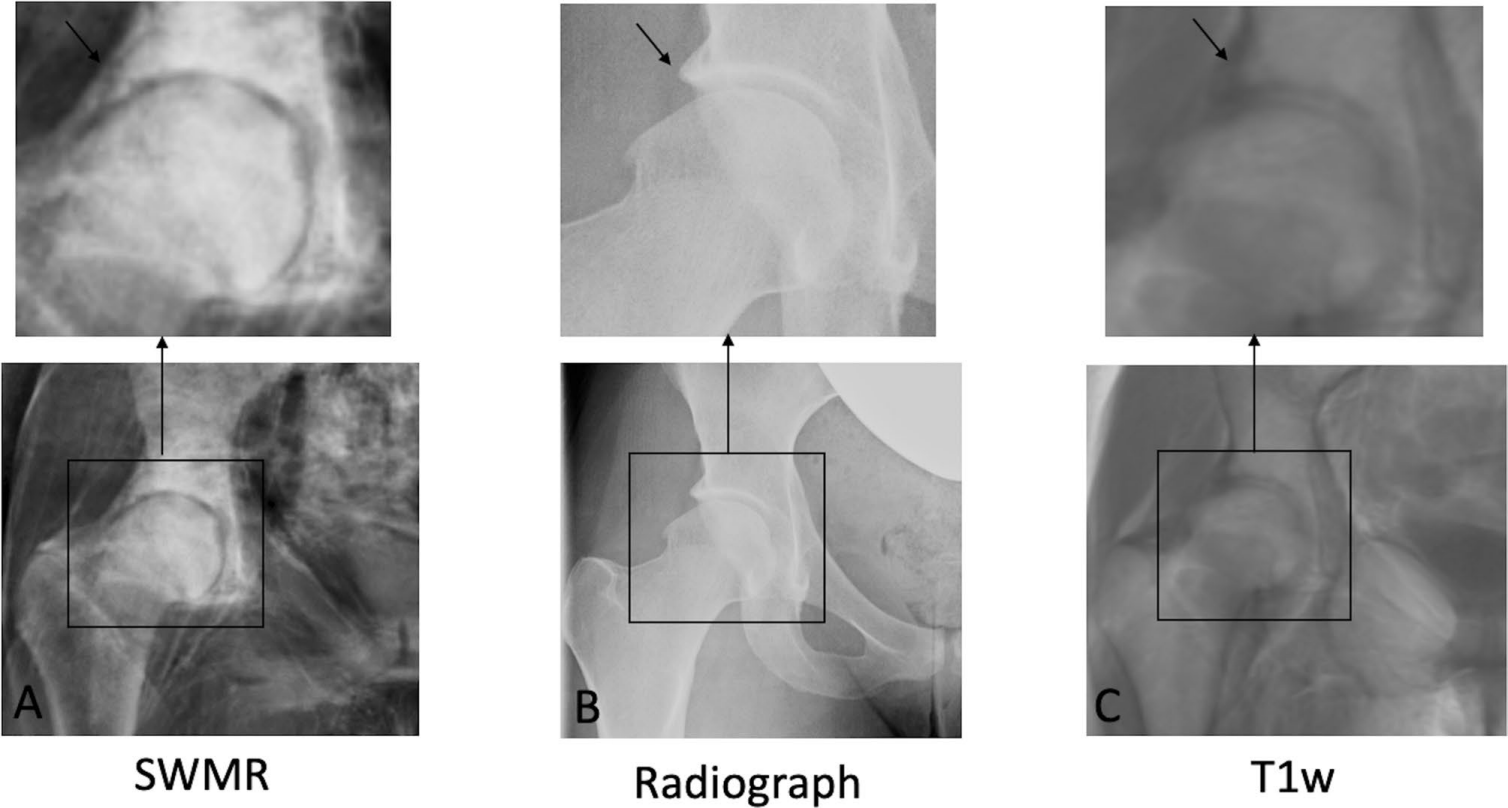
Visibility of the lateral edge of the acetabulum. Radiograph in anteroposterior view (B) and coronal maximum intensity projections of susceptibility-weighted magnetic resonance magnitude images (SWMR; A) and T1weighted magnetic resonance imaging (T1w; C) with magnifications. On SWMR and radiograph, the lateral edge of the acetabulum (arrow) can be identified sharp and clearly. On T1w, the identification of the lateral edge is more difficult and has a blurred shape.
